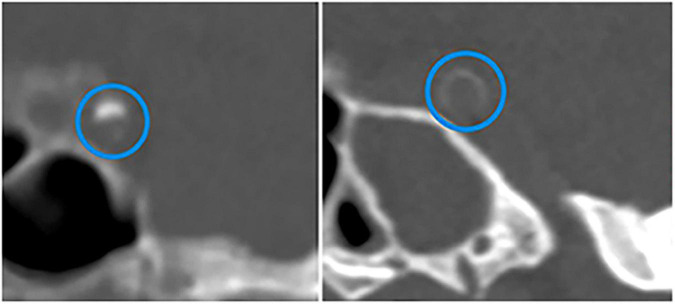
CT images of calcifications in different layers of intracranial internal carotid arteries. Intimal calcification (the left) and medial calcification (the right) are marked by blue circles. Adapted from Kockelkoren et al. (2017).
